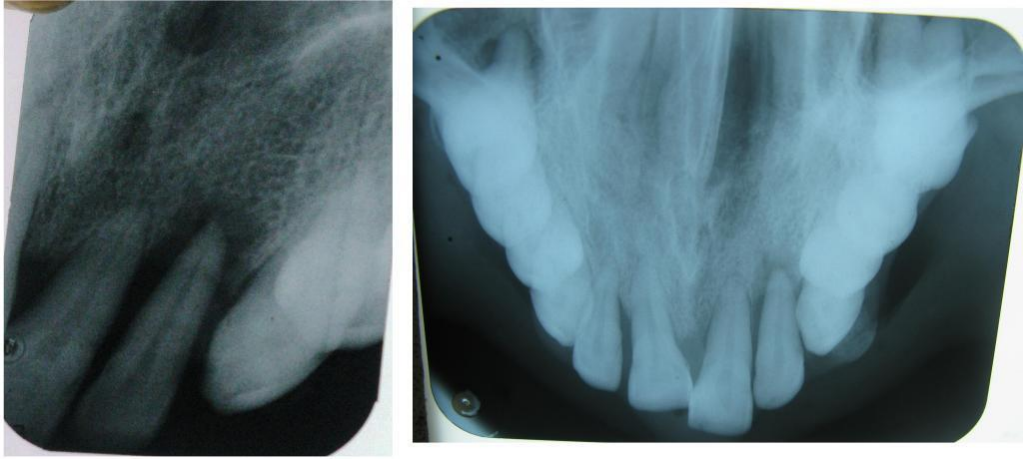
Radiographical representation (IOPAR and maxillary cross-sectional occlusal view
